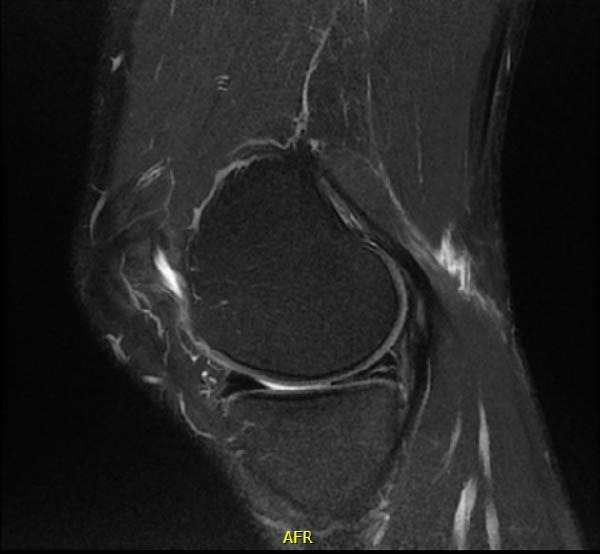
Sagittal MRI cut of right knee (Case 1) showing oblique tear in the posterior horn of medial meniscus.
